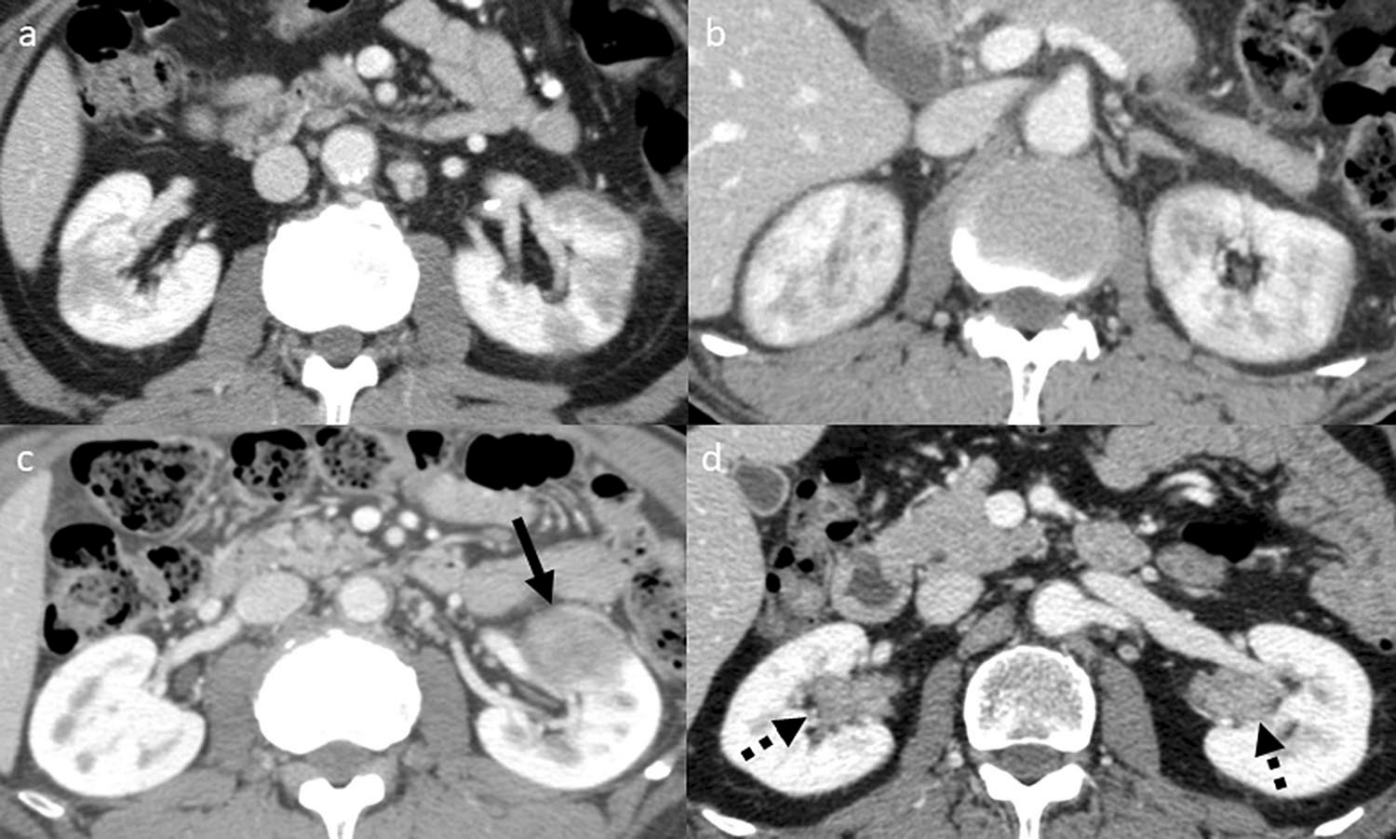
Characteristic findings of IgG4-related kidney disease on contrast-enhanced CT. (a) Multiple hypodense lesions. (b) Diffuse renal swelling. (c) A solitary hypodense lesion (arrow). (d) Diffuse thickening of the renal pelvis wall (broken arrows).
